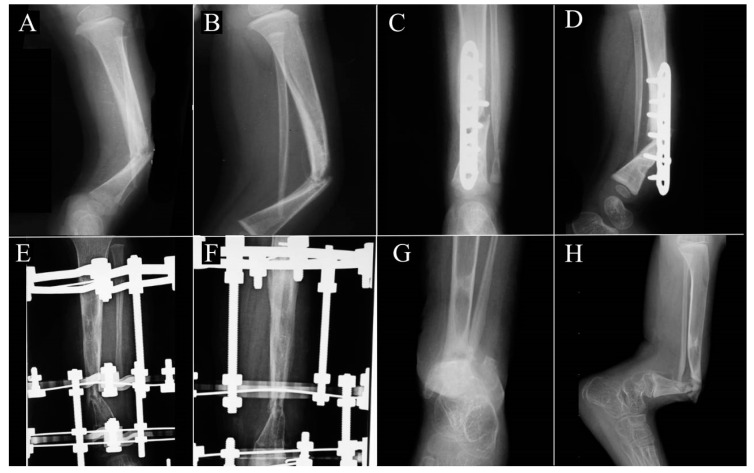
Serial X-rays of patient 2 prior to this correction surgery: (A) anteroposterior (AP) and (B) lateral views of left lower leg at 2 years old; recurrence of deformity (C) AP and (D) lateral views after first correction surgery at age 3; the patient was then placed in an Ilizarov frame, as seen in (E) AP and (F) lateral views, but recurrence occurred at age 4; (G) and (H) show the patient’s deformity at age 11 upon presenting to our institution.
